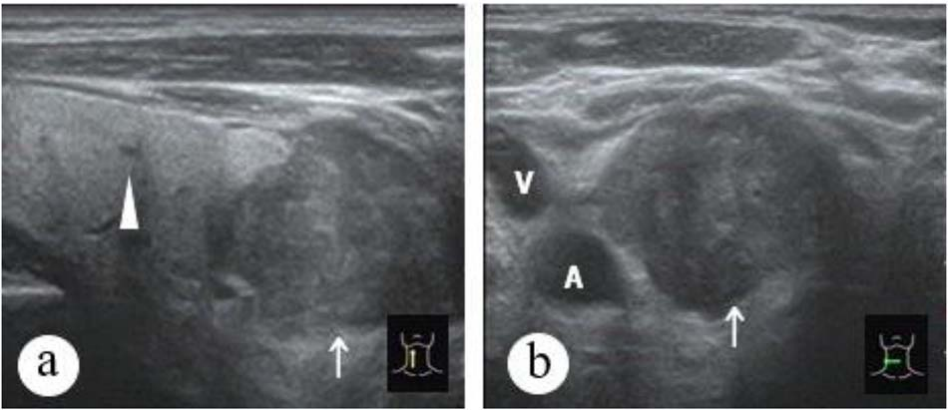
Patient no. 2 (a) thyroid ultrasonography image shows an uneven hypoechoic mass of the tumor, with multiple bands of high echogenicity intersecting inside. CASTLE [→], PTC(
